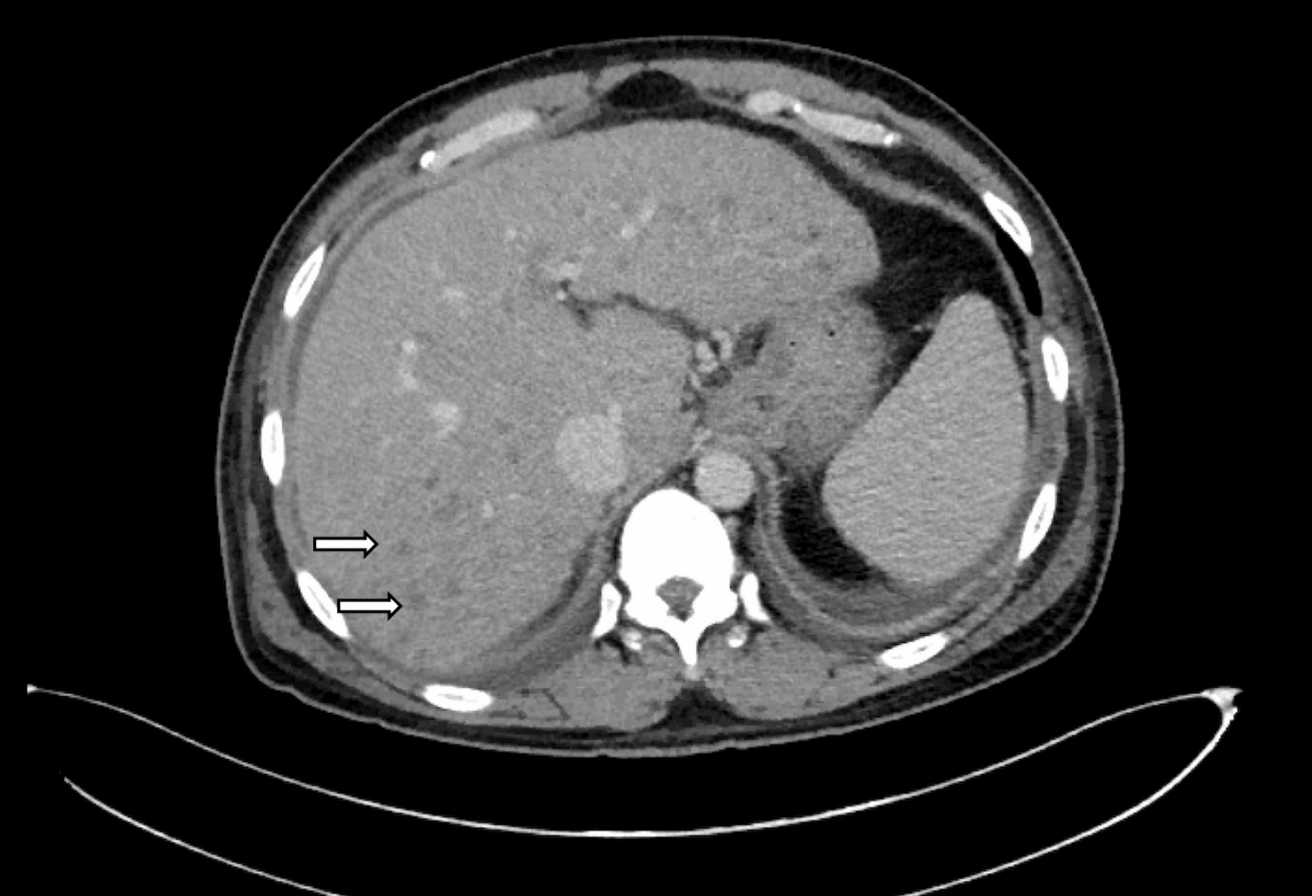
Contrast-enhanced CT abdomen (portal venous phase) shows multiple ring-enhancing lesions throughout the liver (arrows). No central necrosis or washout was evident.
